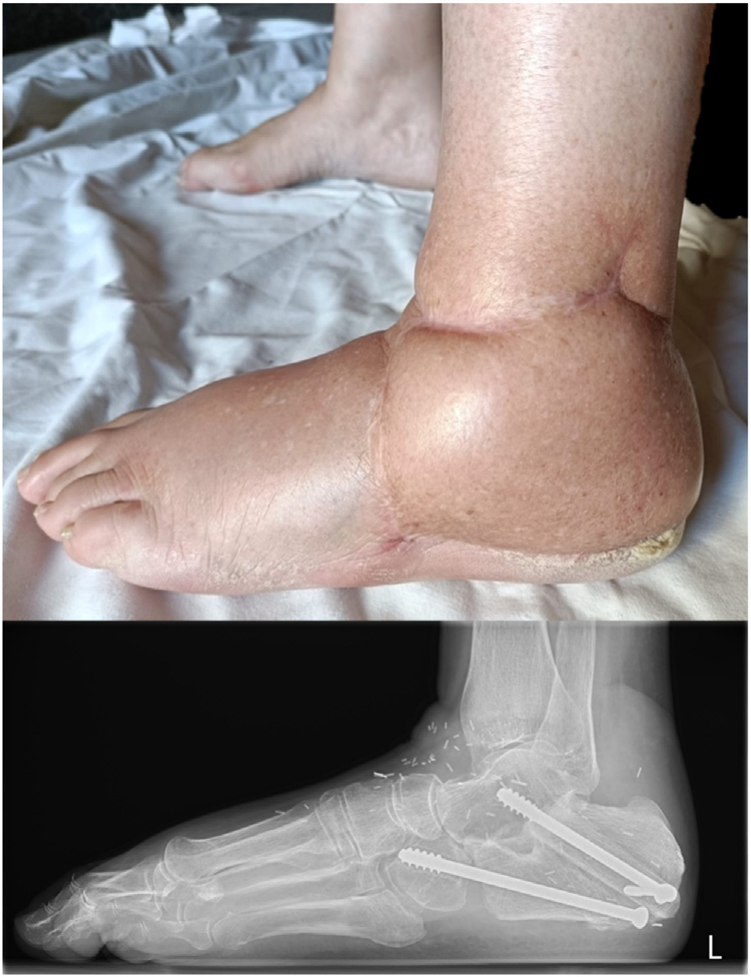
Thirty-six moths of follow up: flap contour (up) and radiography evidencing the fibula bone encased on the allograft, the 3-screw fixation and adequate bone healing (down).
